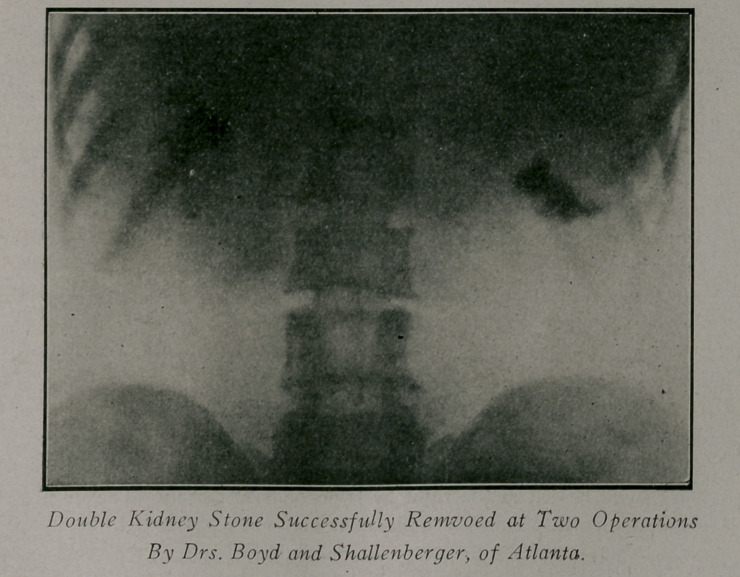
# The Value of the Roentgen Rays in Diagnosis of Kidney Disease
